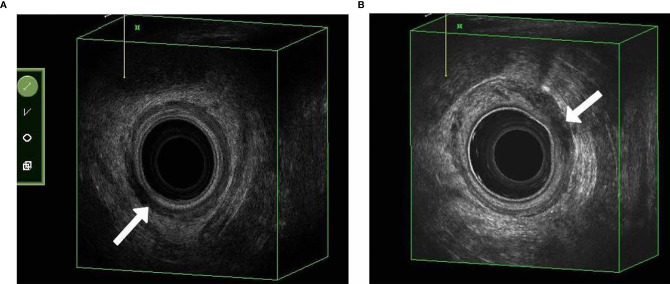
3D-TRUS pictures. (A) A case with complete response determined by three-dimensional transrectal ultrasound (3D-TRUS) and confirmed by TME pathological report (TRG = 0). (B) A case with tumor residue which was also confirmed by TME pathological report with TRG = 3.
